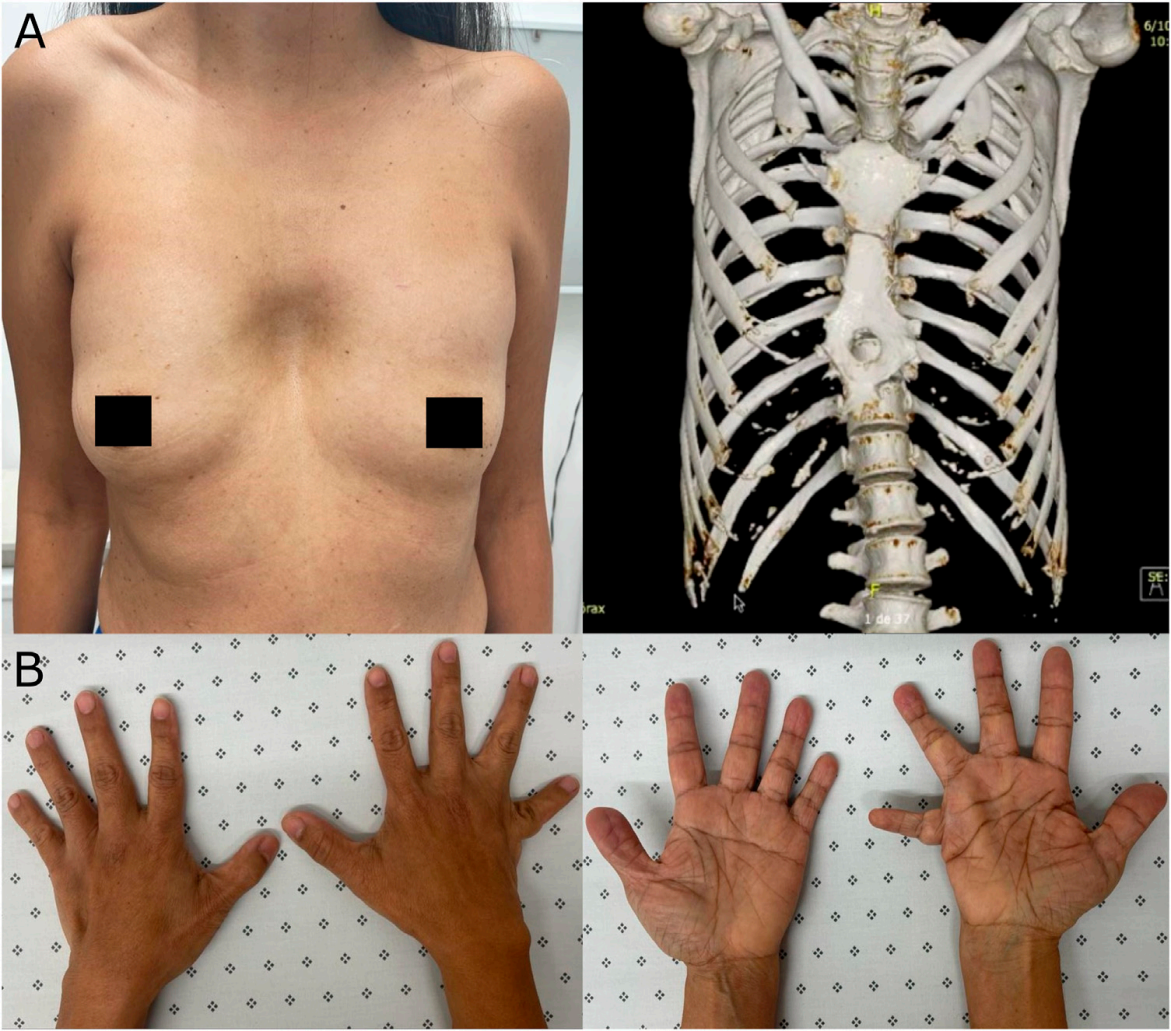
Extracranial manifestations in the patient. (A) Unilateral breast hypoplasia (left) and thorax 3D-CT revealing pectus excavatum (right). (B) Brachydactyly, a low-set fifth finger of the right hand with hypoplastic metacarpal, and a single left transverse palmar crease.
